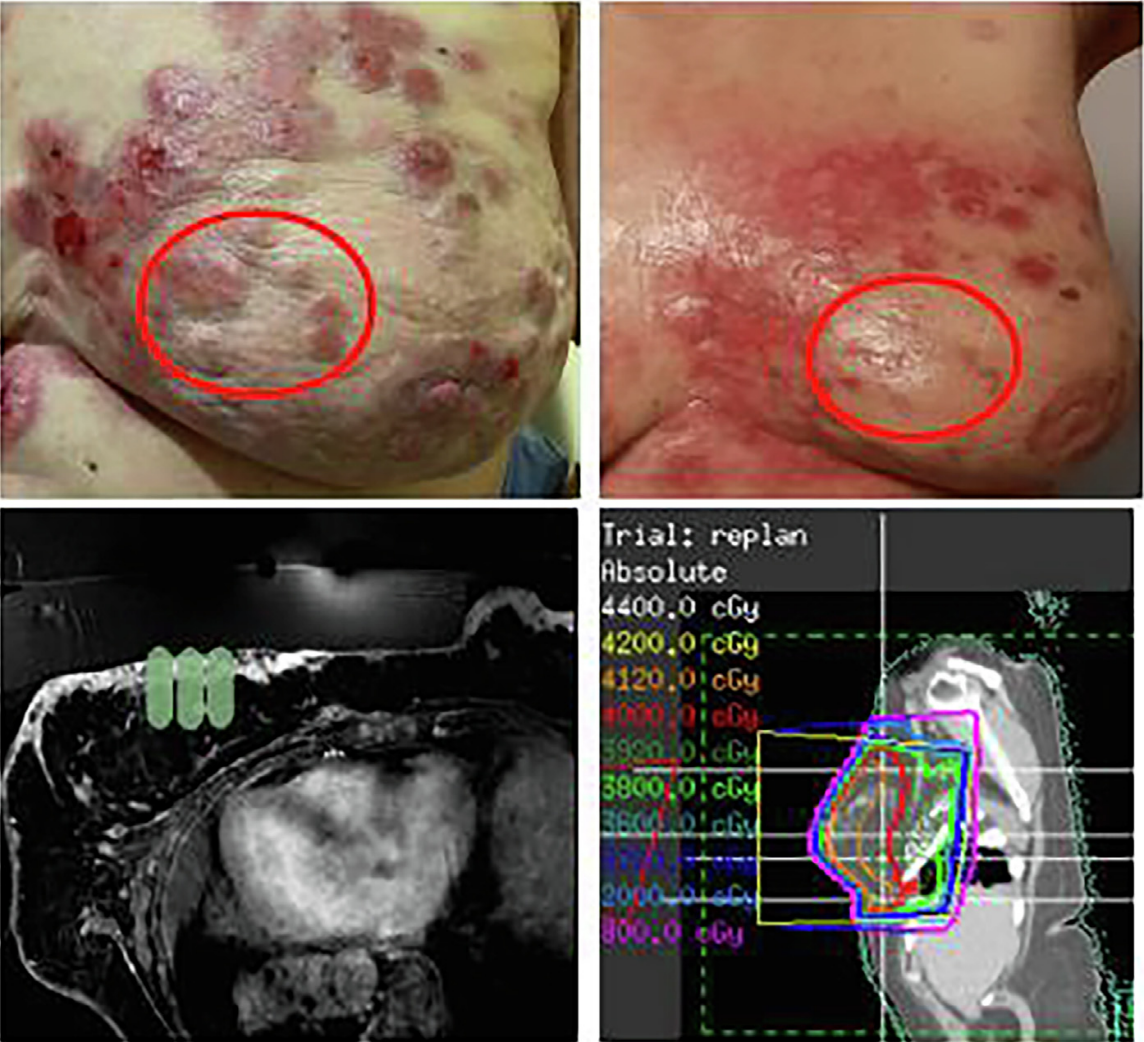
Pilot data from phase I study of ultrasound-microbubble stimulation in breast cancer. Top left: Clinical view of treated lesions before treatment (red ellipse) with MRg-FUS + MB + XRT for radiation enhancement. Ellipse is 5.0 cm in the largest dimension. Top right: Clinical view of lesions indicating diminished lesion sizes 4 weeks after treatment with MRg-FUS + MB. Bottom left: MRI plan indicating where focused ultrasound was directed for microbubble stimulation. Bottom right: MRI plan indicating where radiation was directed for treatment. Treatment with radiation was administered immediately after MRg-FUS + MB therapy From [84].
